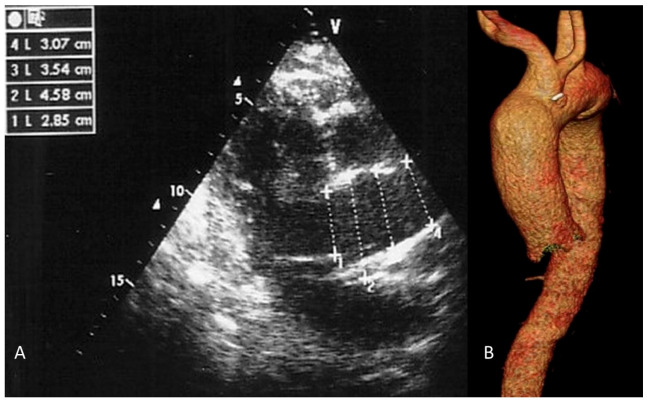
Postoperative imaging assessment at 12 months: (A) Transthoracic echocardiography (parasternal long-axis view) showing a reduced ascending aortic diameter (3.4 cm). (B) CT angiography (multiplanar reconstruction) confirming the stable aortic diameter after reductive aortoplasty.
